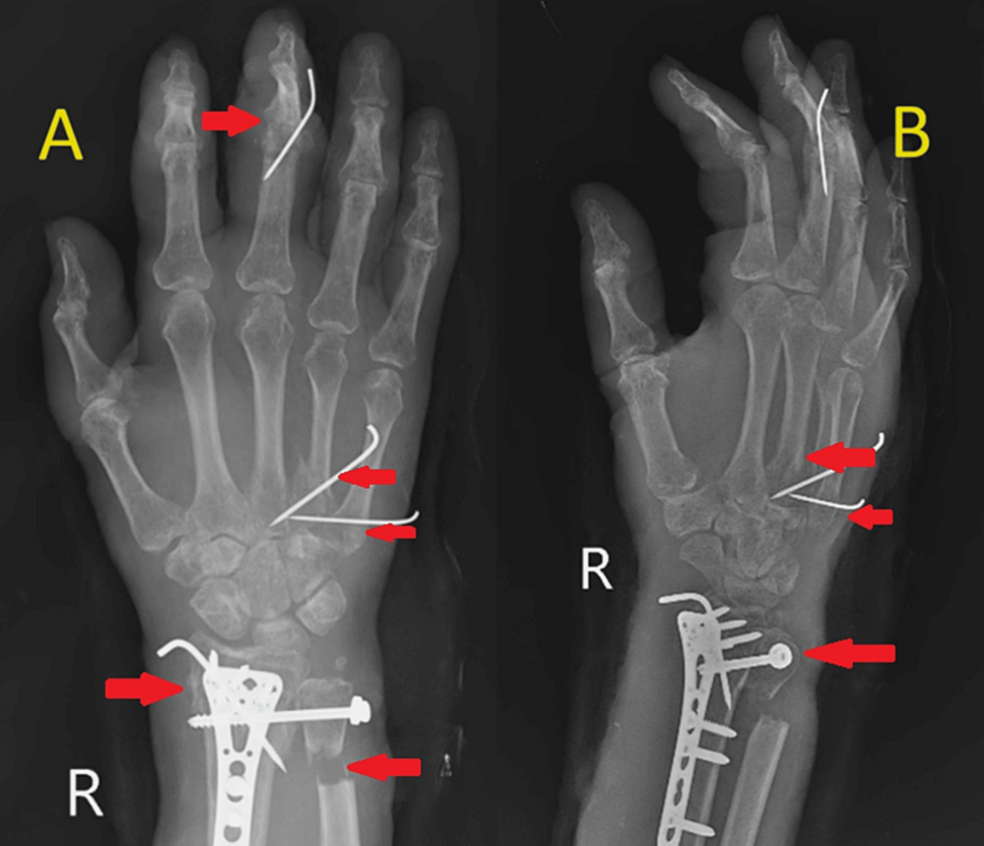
Postoperative X-ray of the right wrist with hand anteroposterior (A) and oblique (B) views (A, B) show closed reduction and internal fixation with a K-wire fixation for the base of the 4^th^ and 5^th^ metacarpal fracture and dislocation of the proximal interphalangeal joint of the 3^rd^ digit of the right hand.
